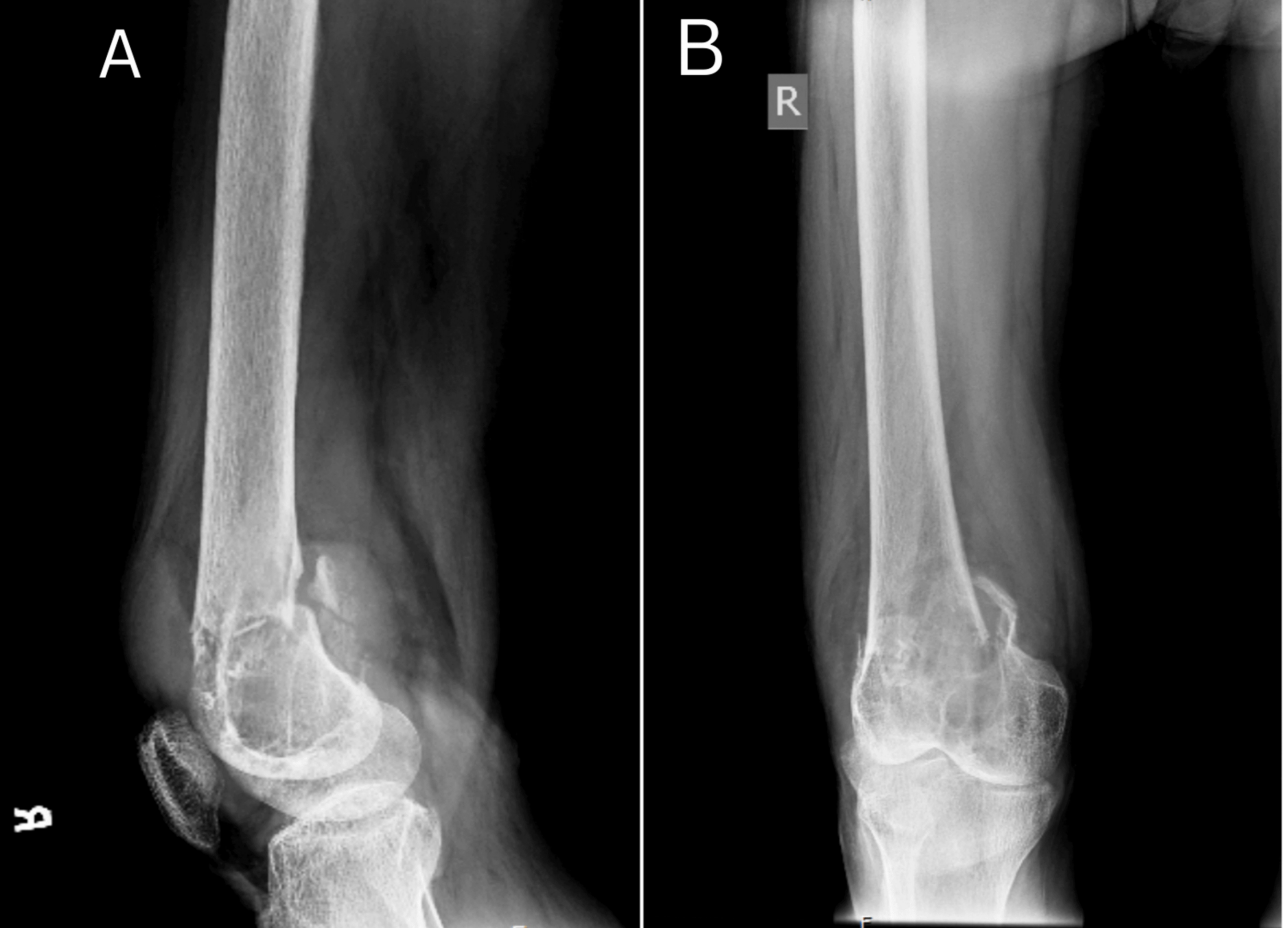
X-ray of the right femur (A) lateral and (B) AP showed a well-defined eccentric lytic lesion seen in the metadiaphyseal region of the distal end of the femur with a fracture of the bony fragment on the medial margin of the femur, which is displaced posteromedially. The lytic lesion involved a fracture of the lateral margin of the femur, leading to overlapping of the bone. No involvement of join margin is seen.
